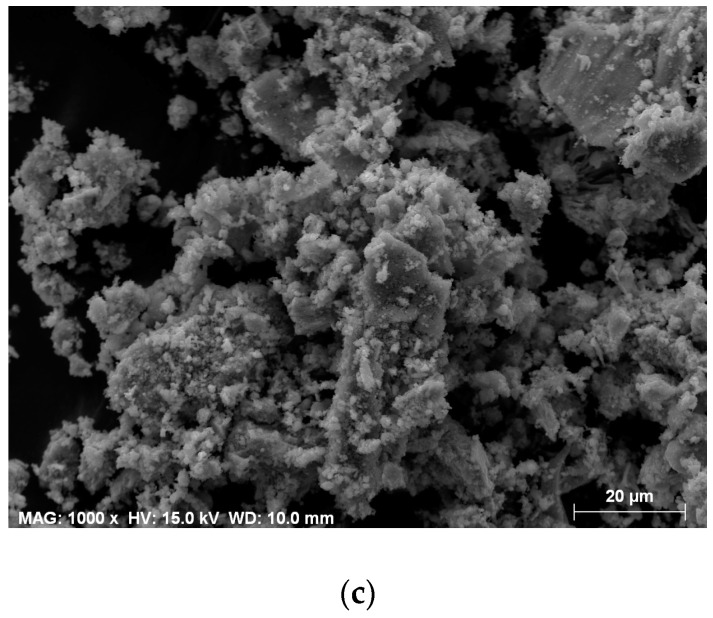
SEM images of the auto-combusted sample (a) energy-dispersive X-ray (EDX) analysis area, (b) the particles after auto-combustion and (c) the sample annealed at 600 °C.
